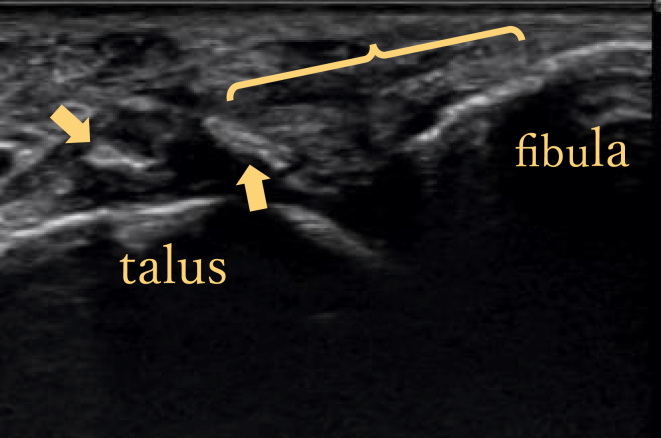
Ultrasonographic findings of anterior talofibular ligament injury site: detached talar bone fragment (arrows) and deflection of anterior talofibular ligament (bracket).
